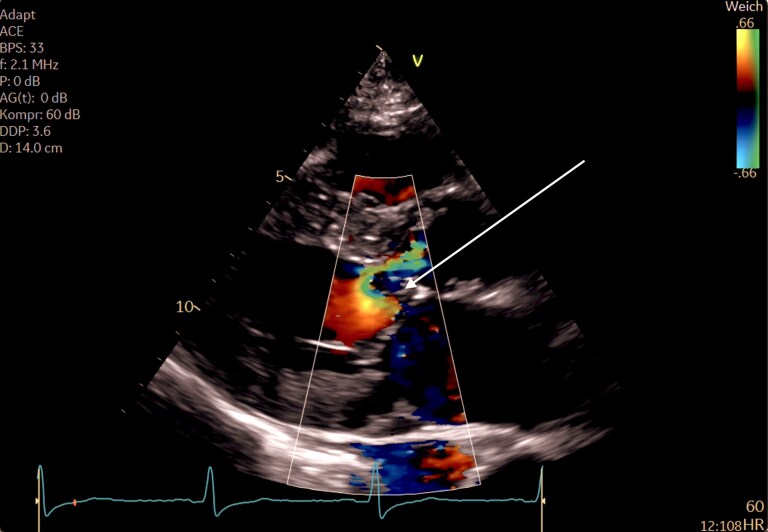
Color Doppler imaging demonstrates pronounced aliasing and flow acceleration within the left ventricular outflow tract, indicating dynamic obstruction without evidence of systolic anterior motion (see Supplementary material online, TTE  *Videos S1–S3*).
